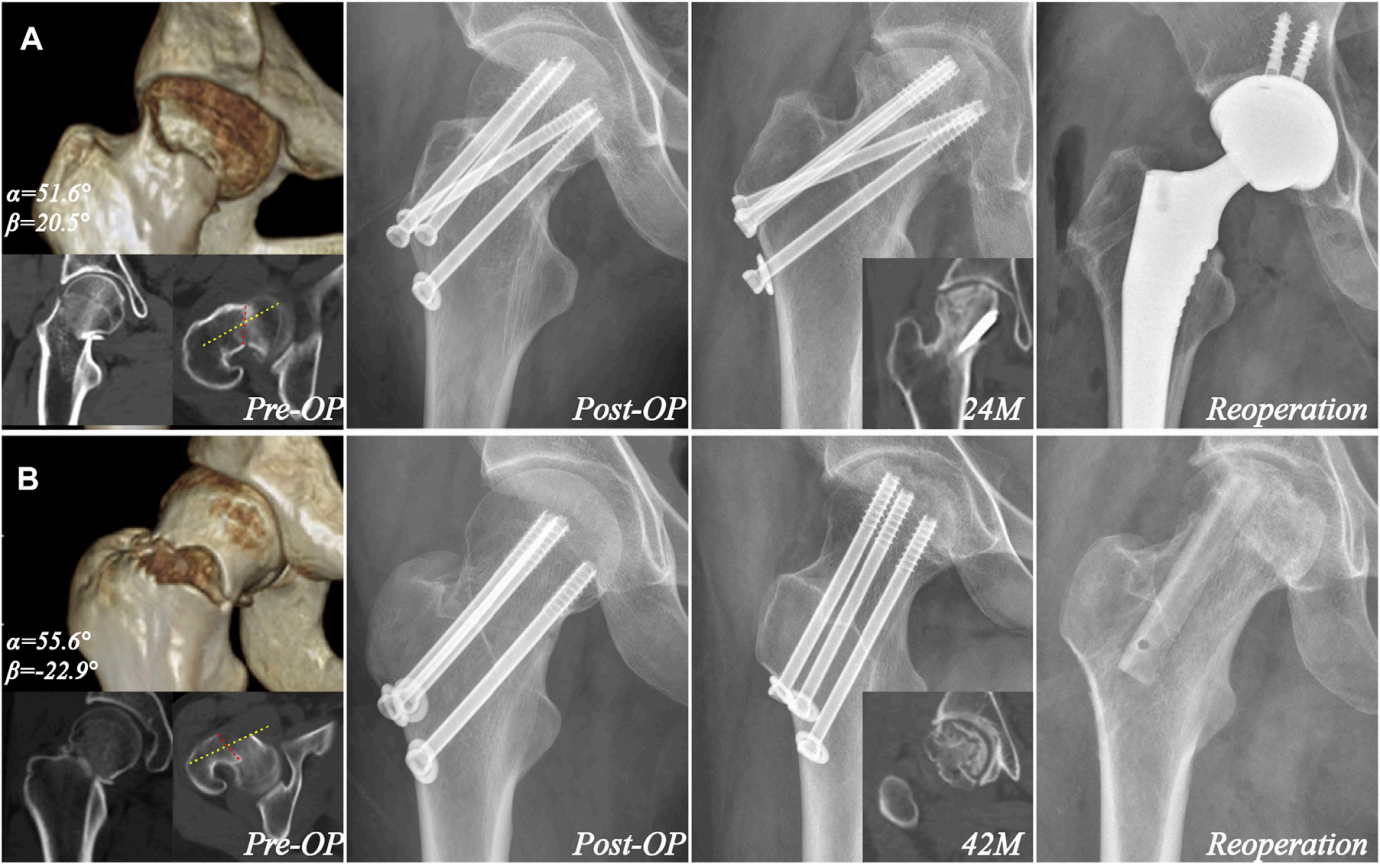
Examples of avascular necrosis in cases with oblique unstable inclination. (A) A 42-year-old female with retroversion oblique unstable inclination angle (α = 51.6° and β = 20.5°) was treated with four cannulated screws. Radiography taken 24 months postoperatively revealed avascular necrosis, which was treated with arthroplasty. The yellow and red line showed the femoral neck axis and fracture orientation in one CT plane. (B) A 32-year-old female with anteversion oblique unstable inclination angle (α = 55.6° and β = −22.9°) was treated with three cannulated screws. Radiography taken 42 months postoperatively revealed avascular necrosis, which was treated with free vascularized fibular grafting. The yellow and red line showed the femoral neck axis and fracture orientation in CT plane.
